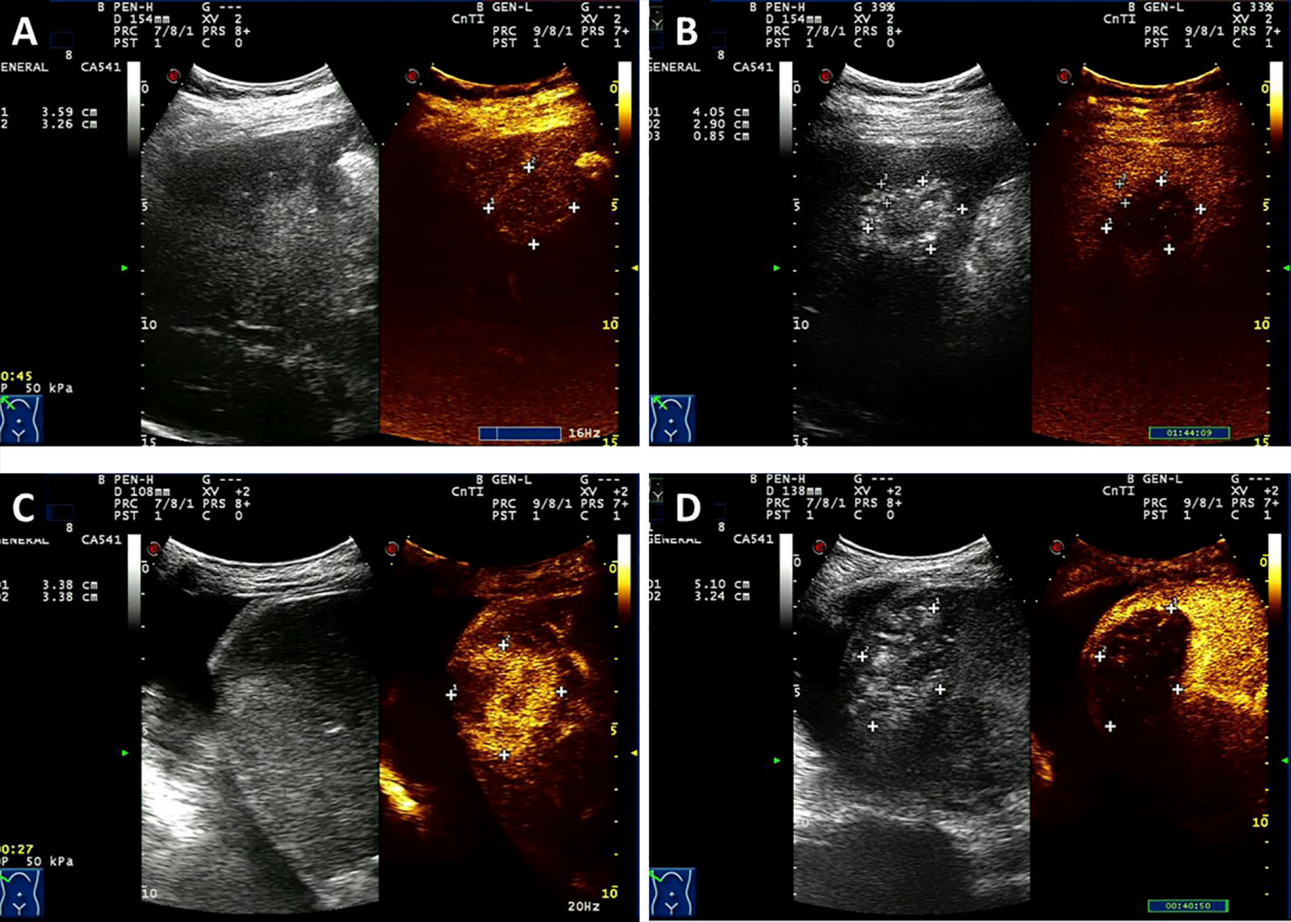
Contrast-enhanced ultrasound (CEUS) of one patient with HCC (A, B) and a case with liver metastases from rectal cancer (C, D) who was treated with HIFU. A patient was newly diagnosed with stage III HCC by puncture biopsy with AFP level > 1000 U/ml and a previous history of hepatitis B. The patient was treated with HIFU without other therapies. (A). Before HIFU ablation, CEUS depicts the enhancing lesion with a richness of blood supply. (B) After treatment, CEUS reveals a completely ablated lesion that shows criteria intratumoral perfusion defect and lacks contrast enhancement. Another patient underwent Dixon surgery and received postsurgery Xelox chemotherapy for six cycles. Twelve months later, hepatic metastatic carcinoma was detected. (C) Before HIFU ablation, CEUS depicts the enhancing lesion with a richness of blood supply. (D) After HIFU ablation, a intratumoral perfusion defect was seen with no contrast enhancement which indicates complete ablation.
